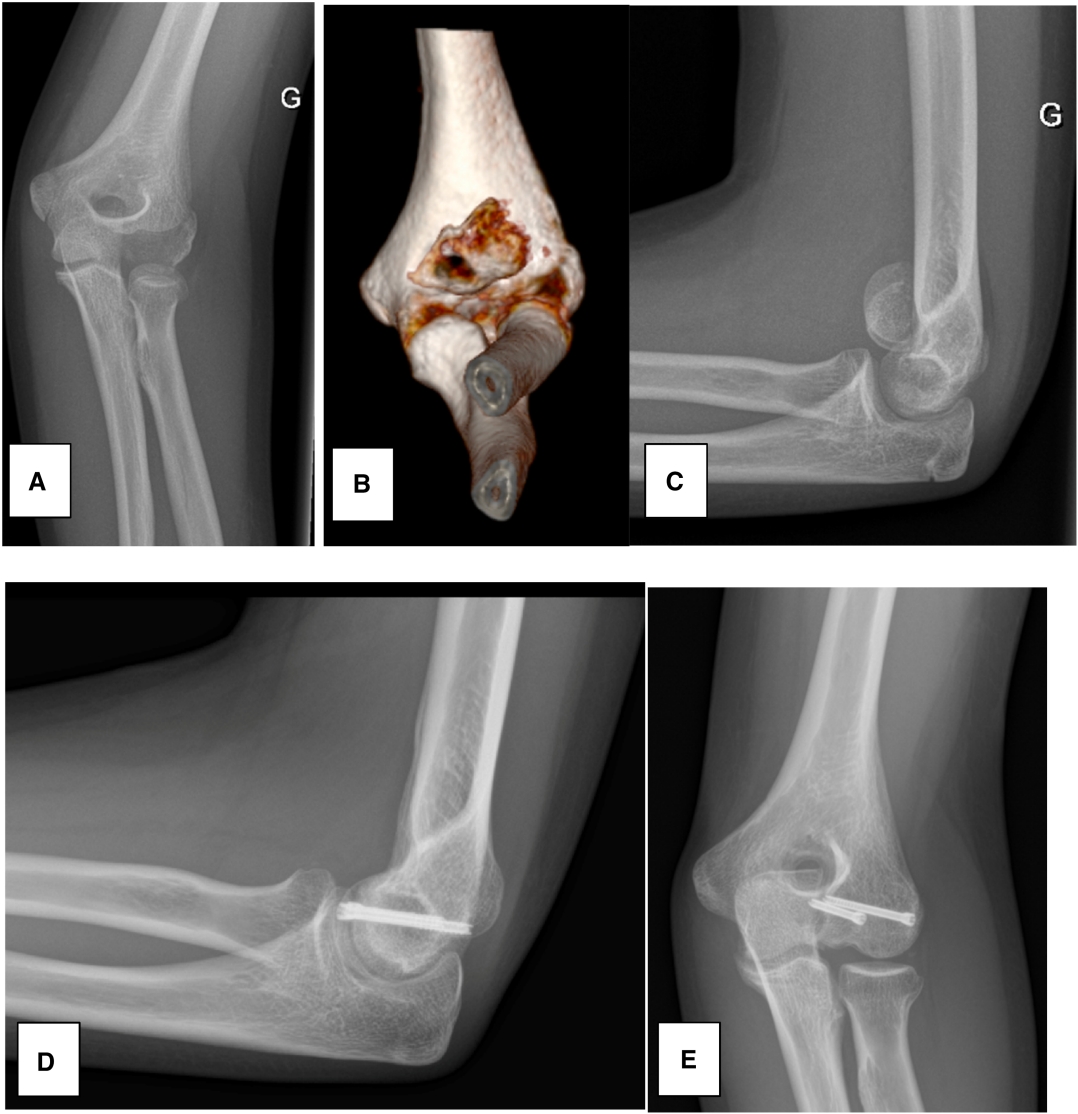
Case 1. (A) AP radiograph at admission. (B) 3D CT-scan showing the capitellar shear fracture, displaced towards proximal and medial. (C) Lateral radiograph at admission, showing the displaced capitellar shear fracture. (D,E) AP and lateral of the 1-year follow-up.
